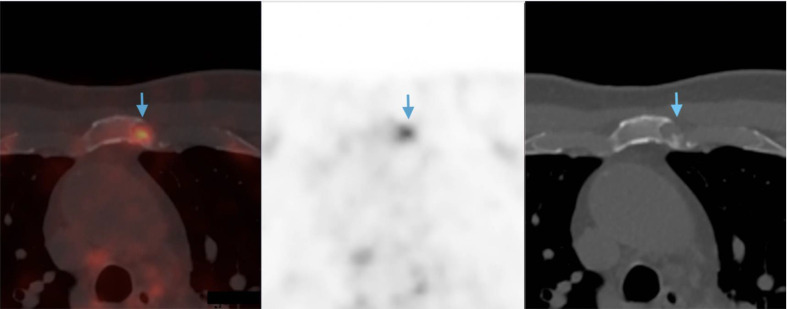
Selected images from the PSMA PET showing mild uptake in the manubrial lesion (blue arrows) (SUVmax of lesion: 6.1) and the CT equivalent showing lytic bone lesion. PET, positron emission tomography; PSMA, prostate-specific membrane antigen; SUVmax, maximum standardised uptake value.
